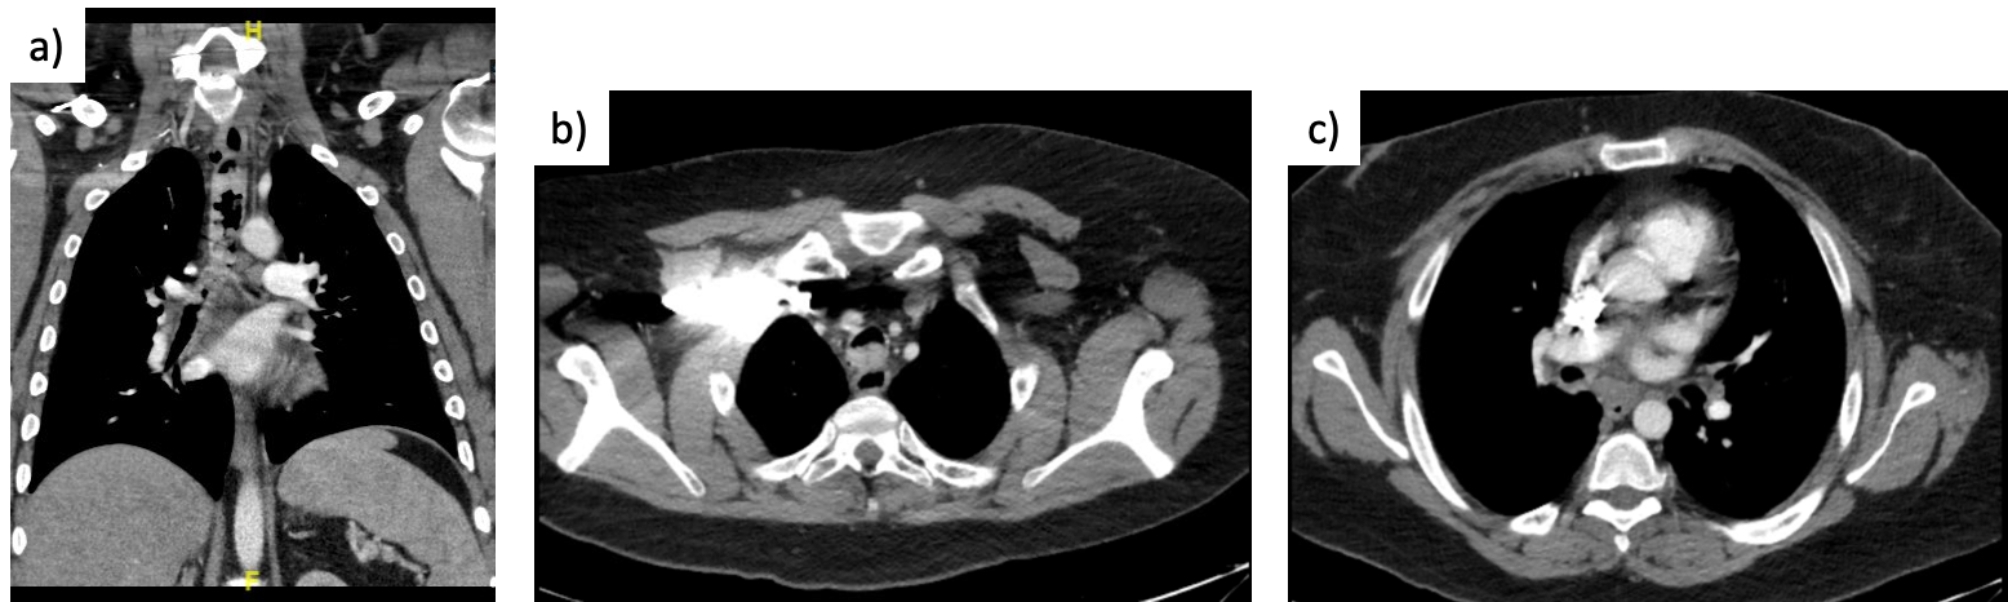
CT scan demonstrating lesions along almost entire length of trachea (a), causing near complete occlusion in proximal trachea (b) and right mainstem bronchus (c)
